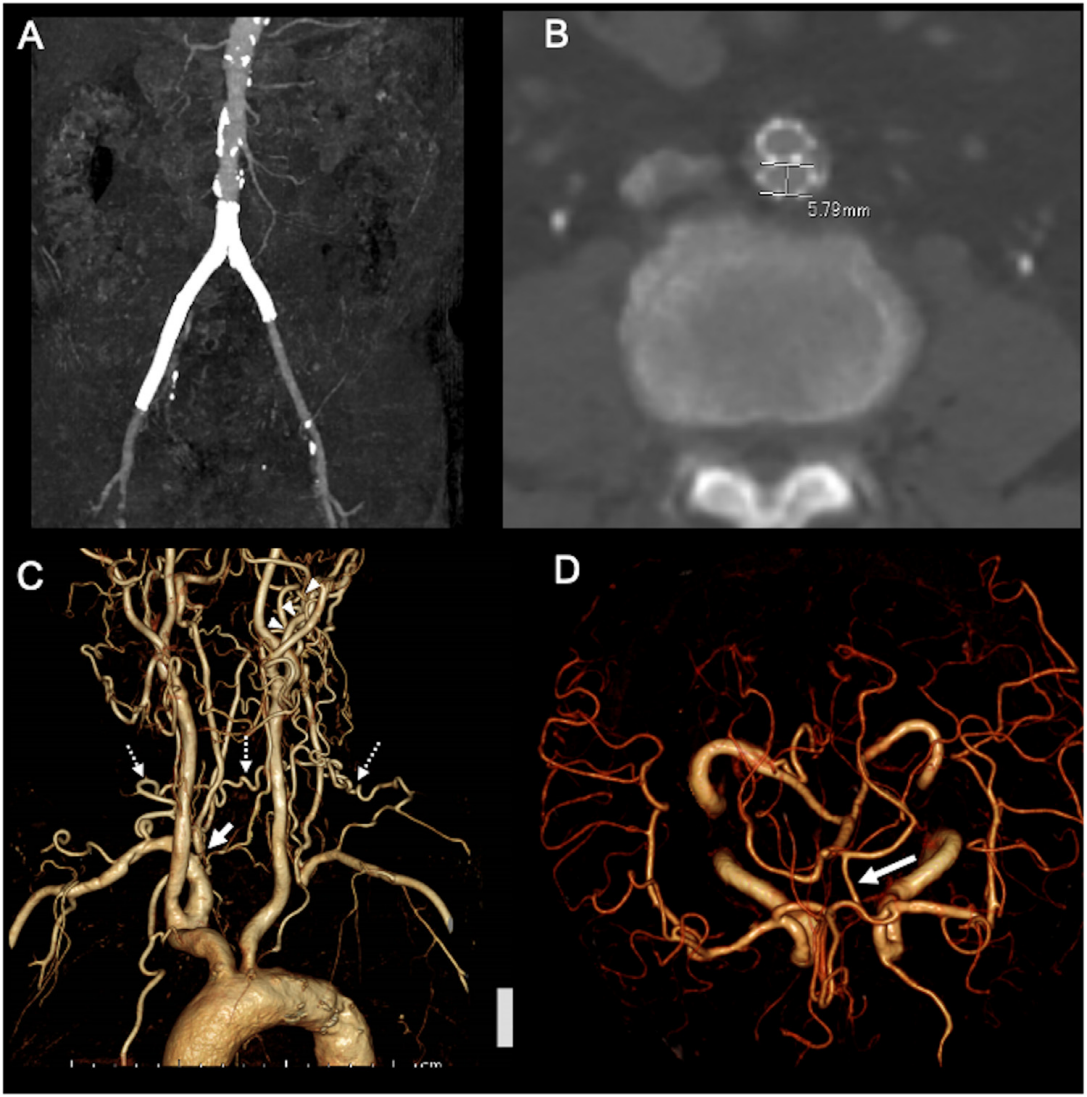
(A) Computed tomography angiography (CTA) demonstrates two stents in both the iliac arteries. (B) Cross-sectional image of the CTA shows the diameter of the stent (5.79 mm), which is larger than the outer diameter of the 9-Fr guiding catheter (3.0 mm). C) CTA of the supra-aortic vessels shows right vertebral artery origin stenosis (arrow), left subclavian artery occlusion, and robust collaterals of the bilateral deep cervical (dotted arrows) and occipital arteries (arrowheads). (D) Intracranial CTA shows communication between the left posterior communicating artery and ipsilateral P1 segment of the posterior cerebral artery.
